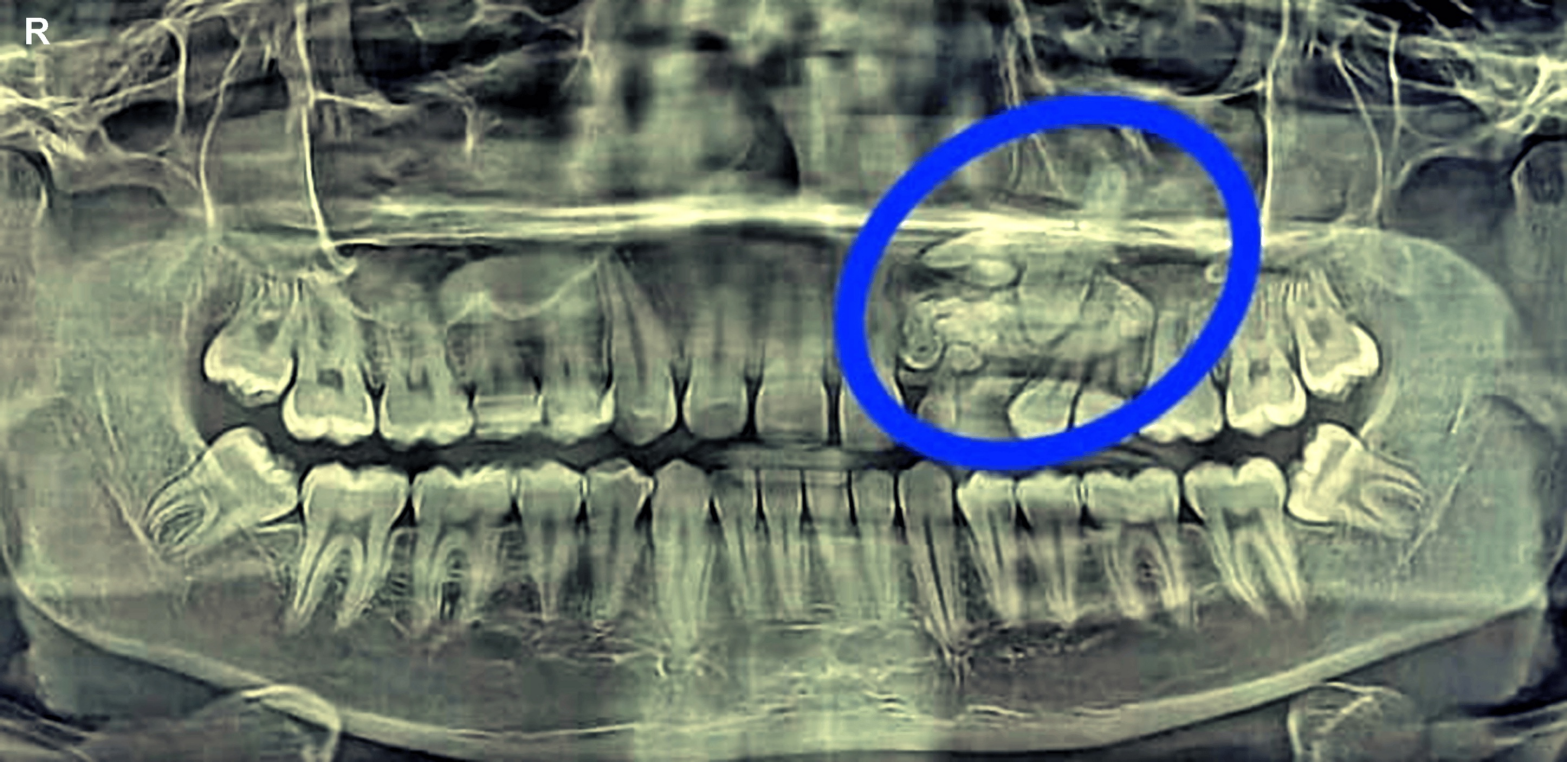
Preoperative OPG of the patient OPG: Orthopantomogram
